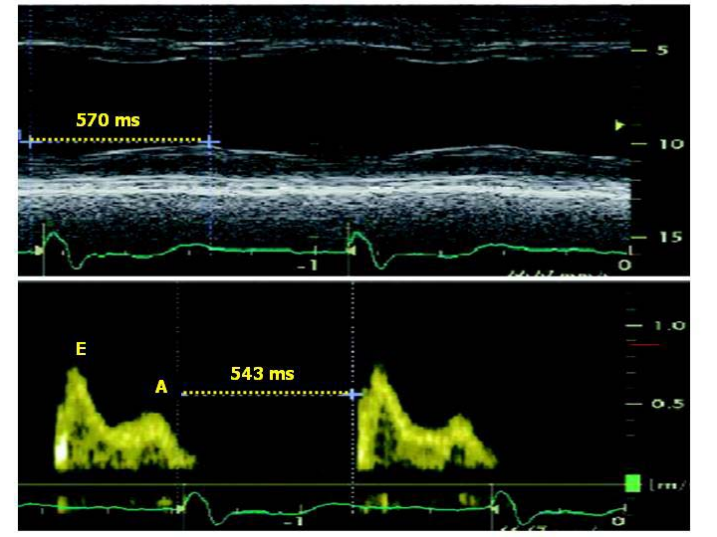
Methodology for measuring lateral wall post-systolic displacement (LWPSD). It is measured as the difference of the time interval from QRS onset to maximal systolic displacement of the basal LV lateral wall (assessed by M-mode in the apical 4-chamber view) (upper panel) and the time interval from QRS onset to the beginning of transmitral E velocity (assessed by pulsed Doppler of mitral inflow) (lower panel). In this example, the positive value of the difference indicates the co-existence of segmental post-systolic contraction and diastolic relaxation. (Modified from Sassone B et al, *Am J Cardiol *2007;100:470–475).
